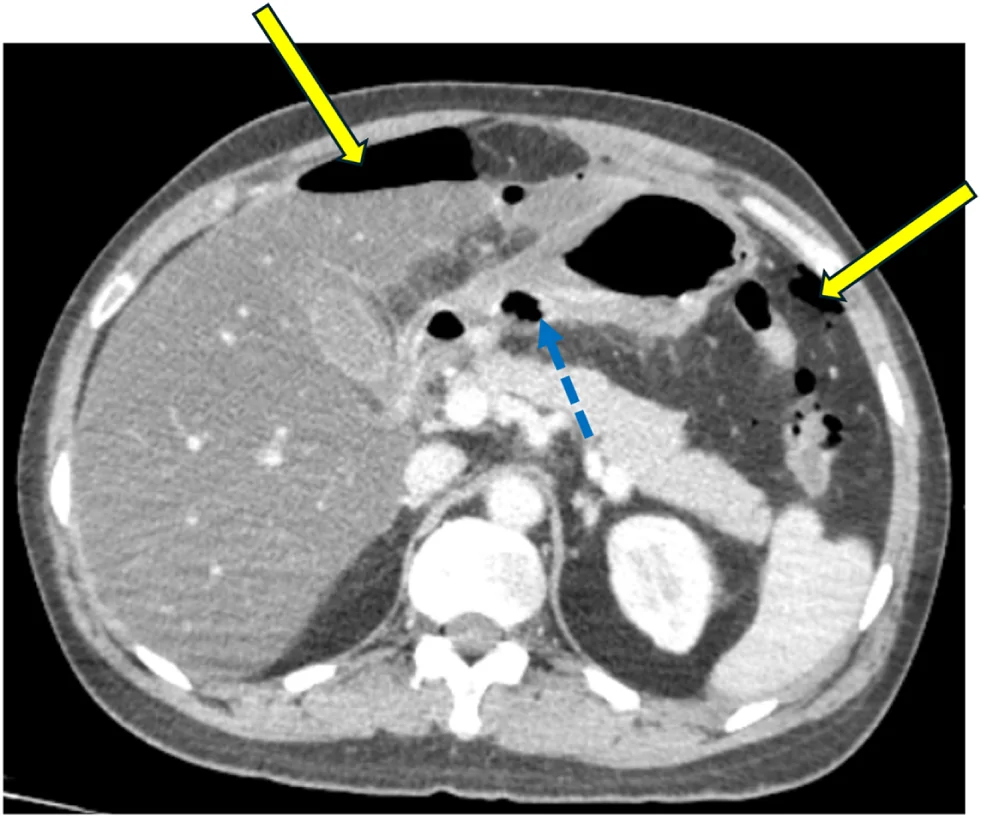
CT scan shows free air (yellow arrows) and a perforation in the stomach (blue dashed – line arrow).
